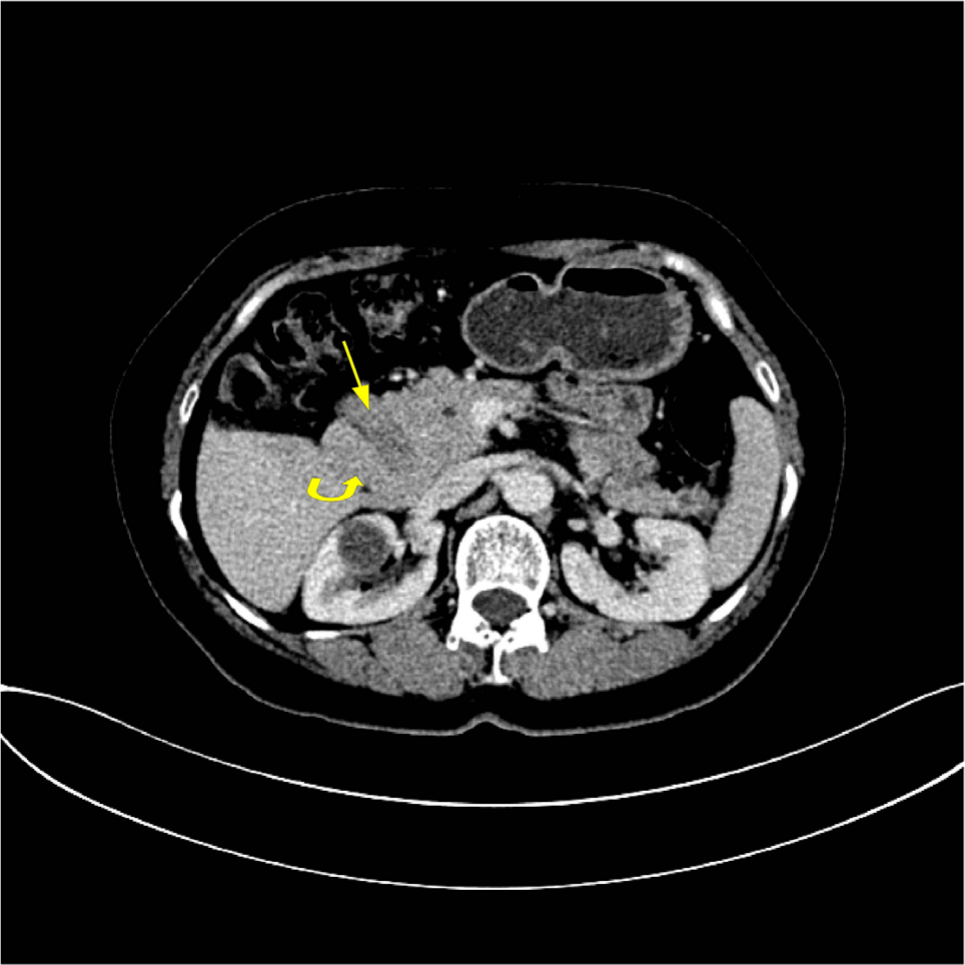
A 50‐year‐old woman with abdominal pain. Axial multiple detector spiral computed tomography (MDCT) venous phase shows duodenum (arrow) completely surrounded by the head of the pancreas (curved arrow), a sandwich sign appearance
